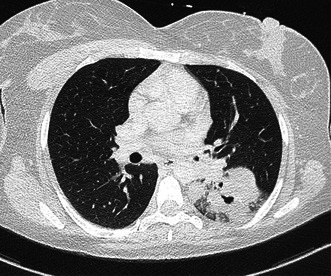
FIGURE 1 CT Chest showing left lower lobe lung mass.
